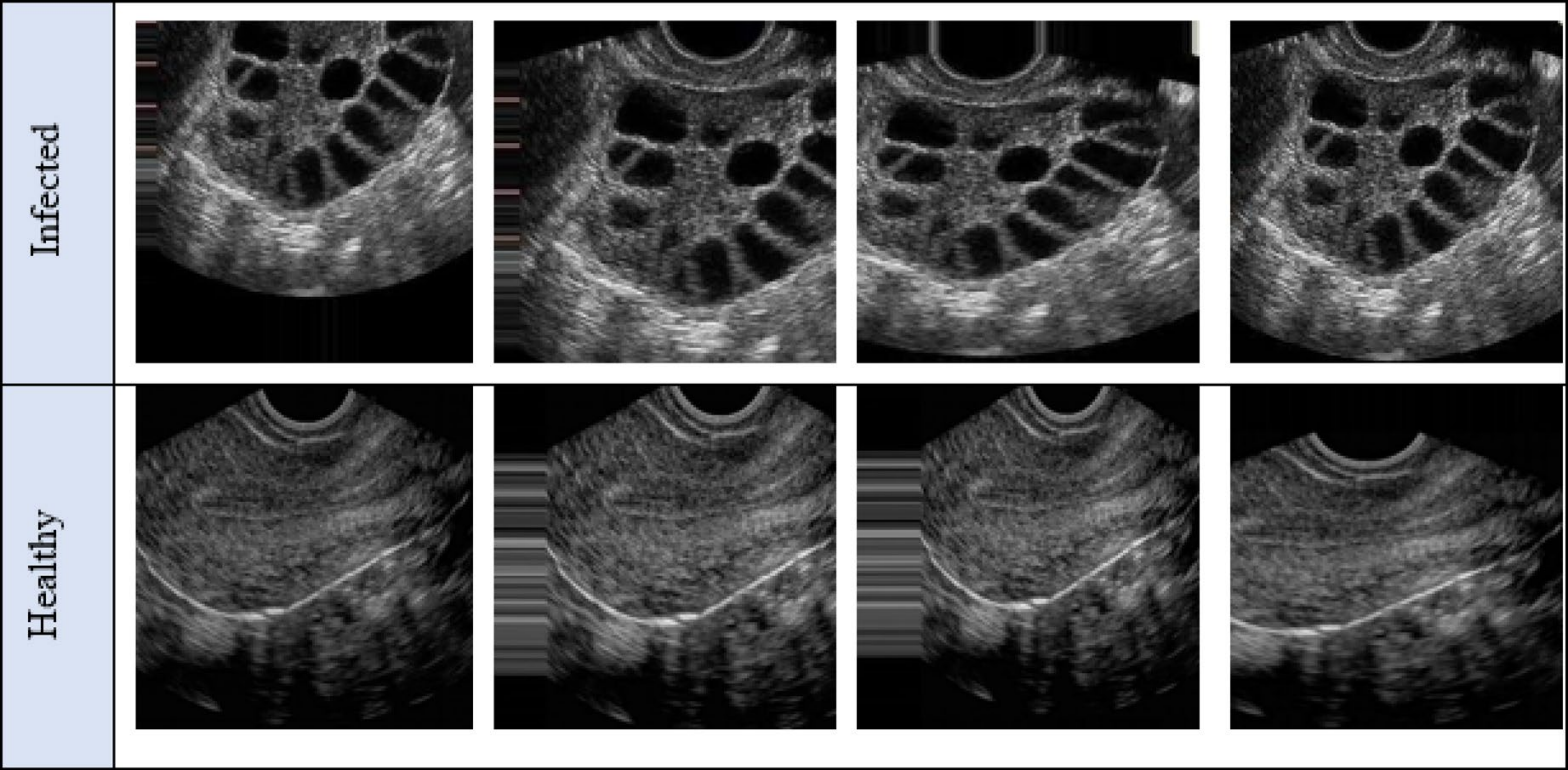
Results of Labeled PCOS Ultrasound Dataset Images.
